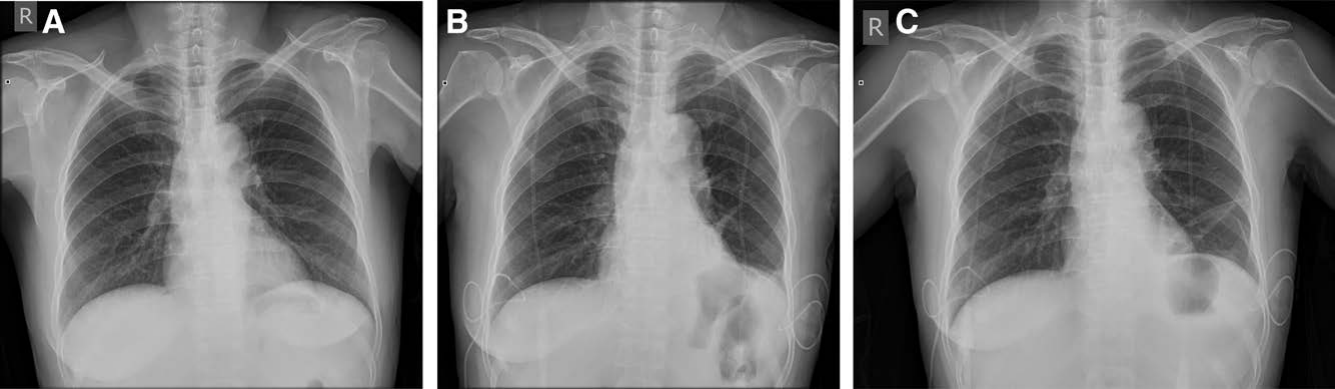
Chest X-rays of case 1: (A) preoperative chest X-ray was normal; (B) postoperative day 4 (POD4) chest X-ray showed lung re-expansion, complete re-expansion of the right lung, and indistinct left costophrenic angle, with bilateral pigtail catheters placed (arrows); (C) predischarge chest X-ray on POD9 after administration of octreotide showed bilateral lung re-expansion and sharp bilateral costophrenic angles (arrows).
